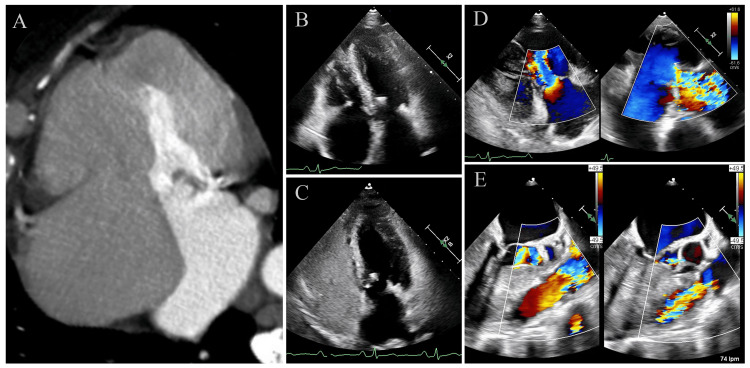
Carcinoid-related valvular heart disease (A) Axial, arterial-phase contrast-enhanced computed tomography images, showing marked dilation of the right-sided heart chambers with flattening of the interventricular septum and leftward displacement of the interatrial septum secondary to right-sided strain, as well as calcification of the mitral valve and annulus. (B–C) Transthoracic echocardiography images in the apical four-chamber view during early diastole, showing right chamber dilation and mitral-tricuspid valvular degeneration, with extensive carcinoid-related calcification of both valves and the right subvalvular apparatus, as well as no bubble passage to the left chambers. (D–E) Transesophageal echocardiography images with color Doppler in the mid-esophageal view of the tricuspid valve (105°) and pulmonary valve (120°), both demonstrating mild opening restriction and severe regurgitation.
